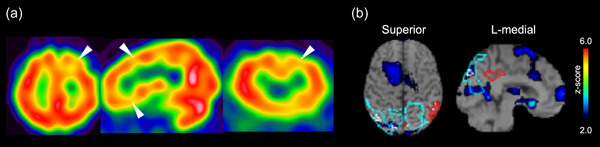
Cerebral perfusion single photon emission computed tomography (SPECT) images of Case A. (a) Images of brain perfusion SPECT of Case A. Colored areas show significantly increased (red) or decreased (blue) perfusion compared with (b) age‐matched controls.
